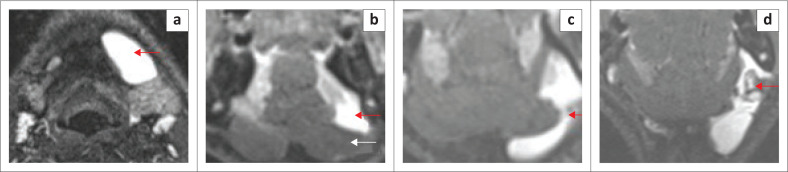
A plunging (diving) ranula in an adult patient with a history of a fluctuant soft swelling in the left submandibular region. Initial ultrasound (not included) revealed a partly imaged cystic lesion. T2 axial MRI neck (a) indicates a cystic lesion in the left submandibular region, anterior to the left submandibular gland, extending from anterior floor of the mouth. The T2-weighted short tau inversion recovery (T2W-STIR) coronal anterior image (b) shows the anterior extent of the cyst in the left sublingual space (red arrow) above the anterior belly of digastric muscle (b, white arrow). The mid part of lesion (c) shows the cyst is dissecting inferiorly, deep to mylohyoid muscle (red arrow), suggesting a tail-sign. The T2-weighted short-tau inversion recovery (T2W-STIR) coronal image (d) shows small non-cystic debris (red arrow); there was no evidence of a vascular malformation.
